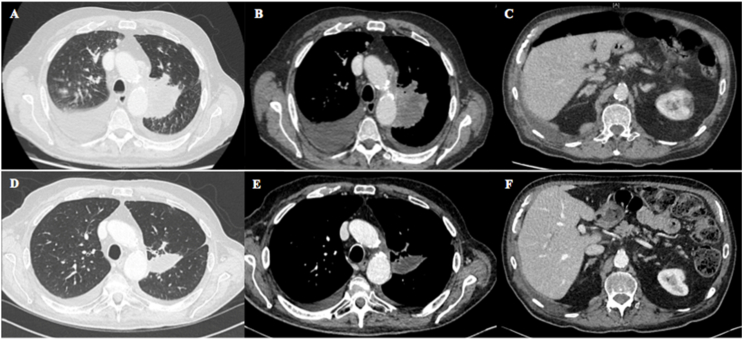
A-C: Baseline CT scan; D-F: CT scan performed 2 months after crizotinib initiation.
